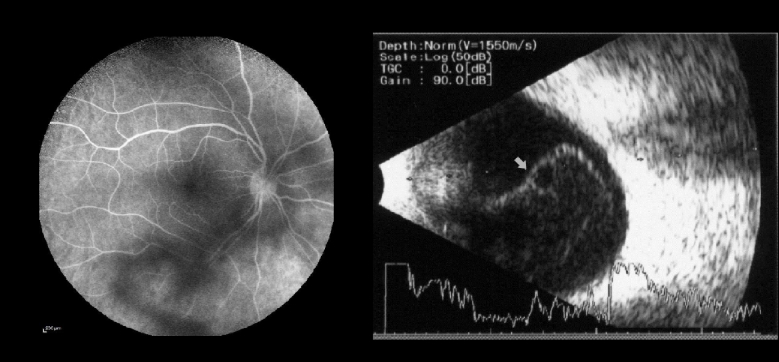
Left: Fluorescein angiography during the arteriovenous phase shows a hot disc and hypofluorescent amoeboid shadow of the detached vitreous body. Right: B-scan ultrasonography (gain: 90 dB) shows the detached vitreous gel encompassing numerous fine reflective opacities and a thickened echogenic membrane in the peripheral vitreous compartment (arrow).
